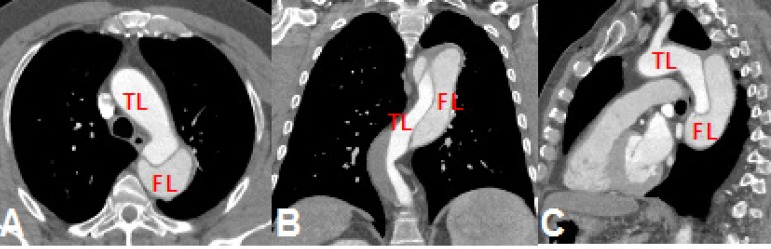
Case 5: computed tomography (CT) angiography of aortic dissection
